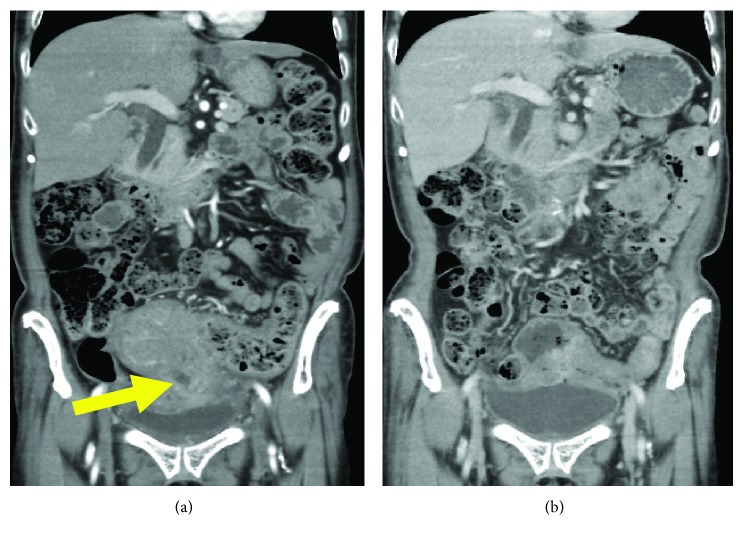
Contrast-enhanced CT showed an irregular mass with infiltration into the bladder. The arrow indicates the communication between the mass and the bladder (a). After 5 courses of neoadjuvant chemotherapy, CT scan revealed shrinkage of the primary tumor (b).
